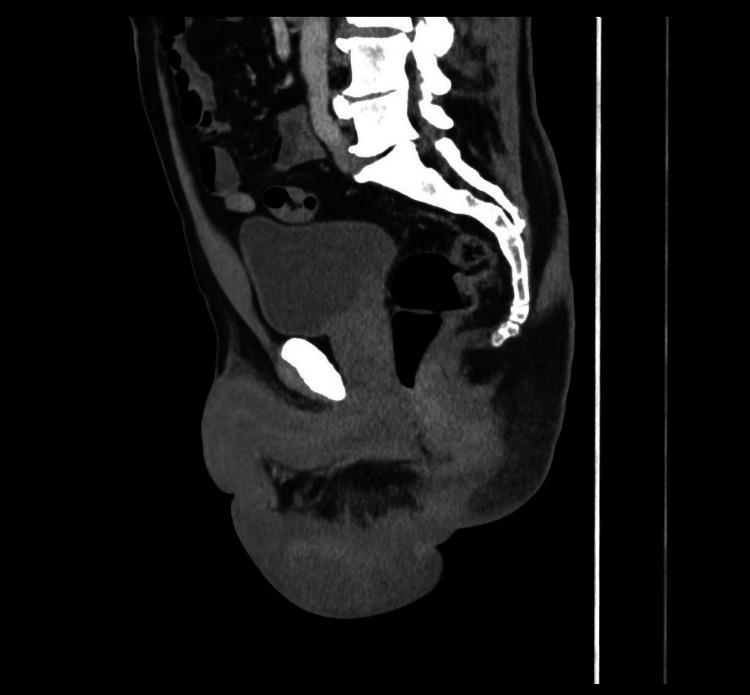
Computed tomography demonstrating soft tissue inflammation extending into the perineum.
